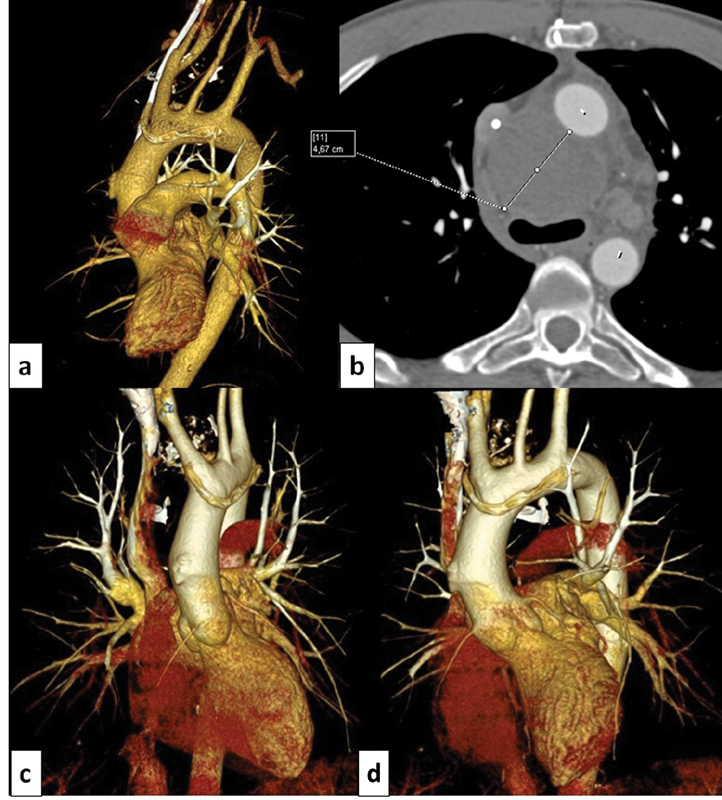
Postoperative CT of the operation zone 15 days after surgery (
